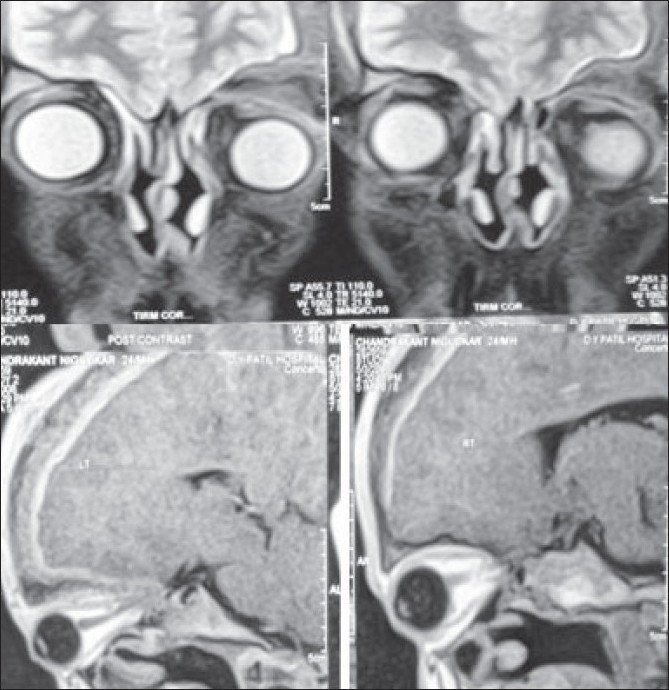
Magnetic resonance imaging showing dystopia and increase in left orbit volume
